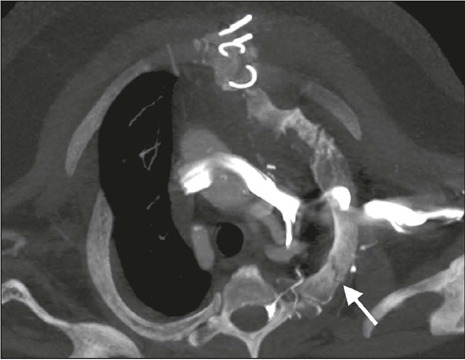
Rib fracture (axial oblique CT image; maximum intensity projection
